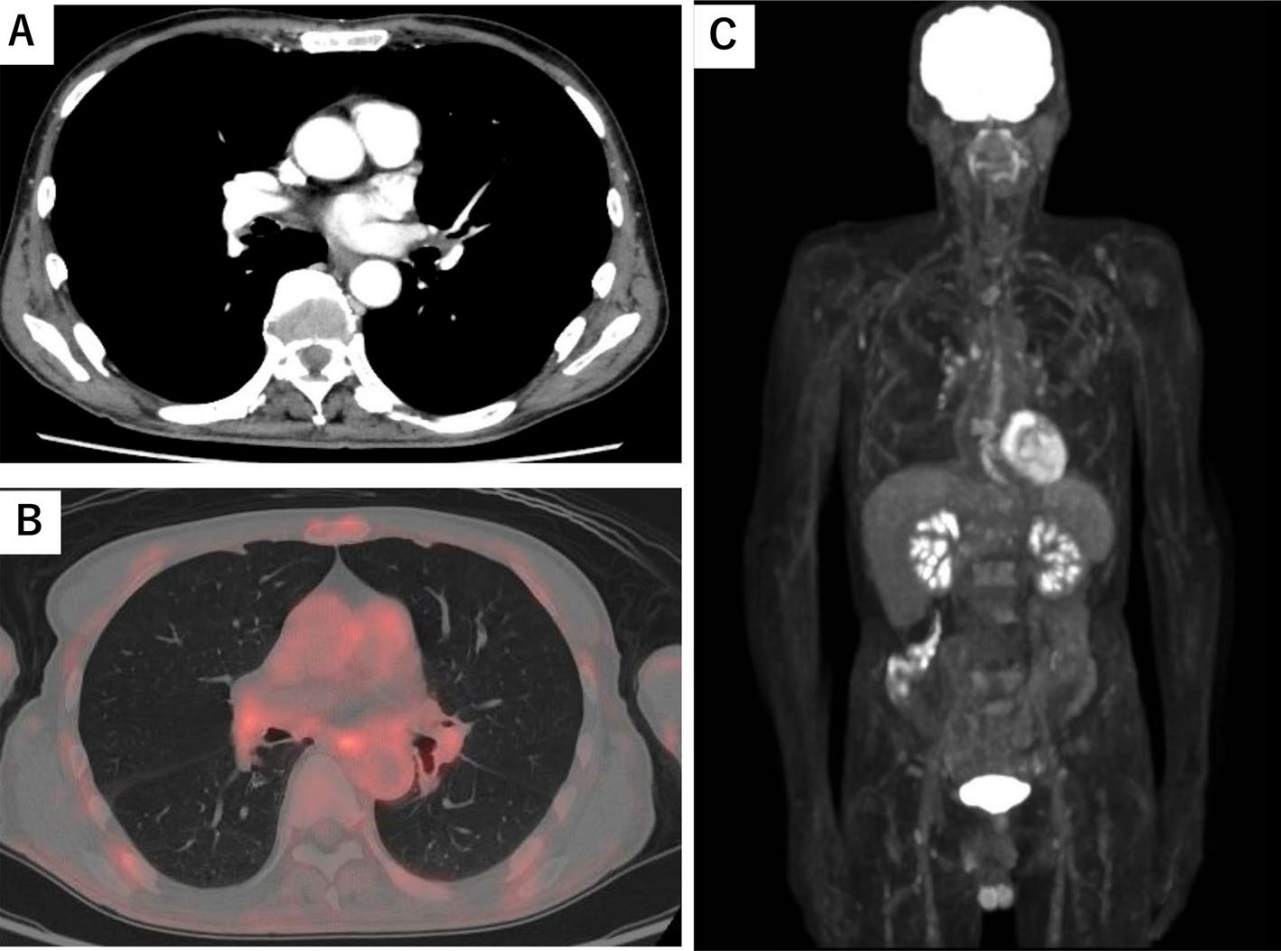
Radiographic findings after immunochemotherapy. (A) Chest computed tomography revealed resolution of left main bronchial wall thickening and left hilar lymph node swelling after immunochemotherapy. (B, C) Positron emission tomography with fluorodeoxyglucose‐computed tomography (FDG‐PET/CT) showed complete resolution of FDG uptake in all previously identified lesions after immunochemotherapy.
